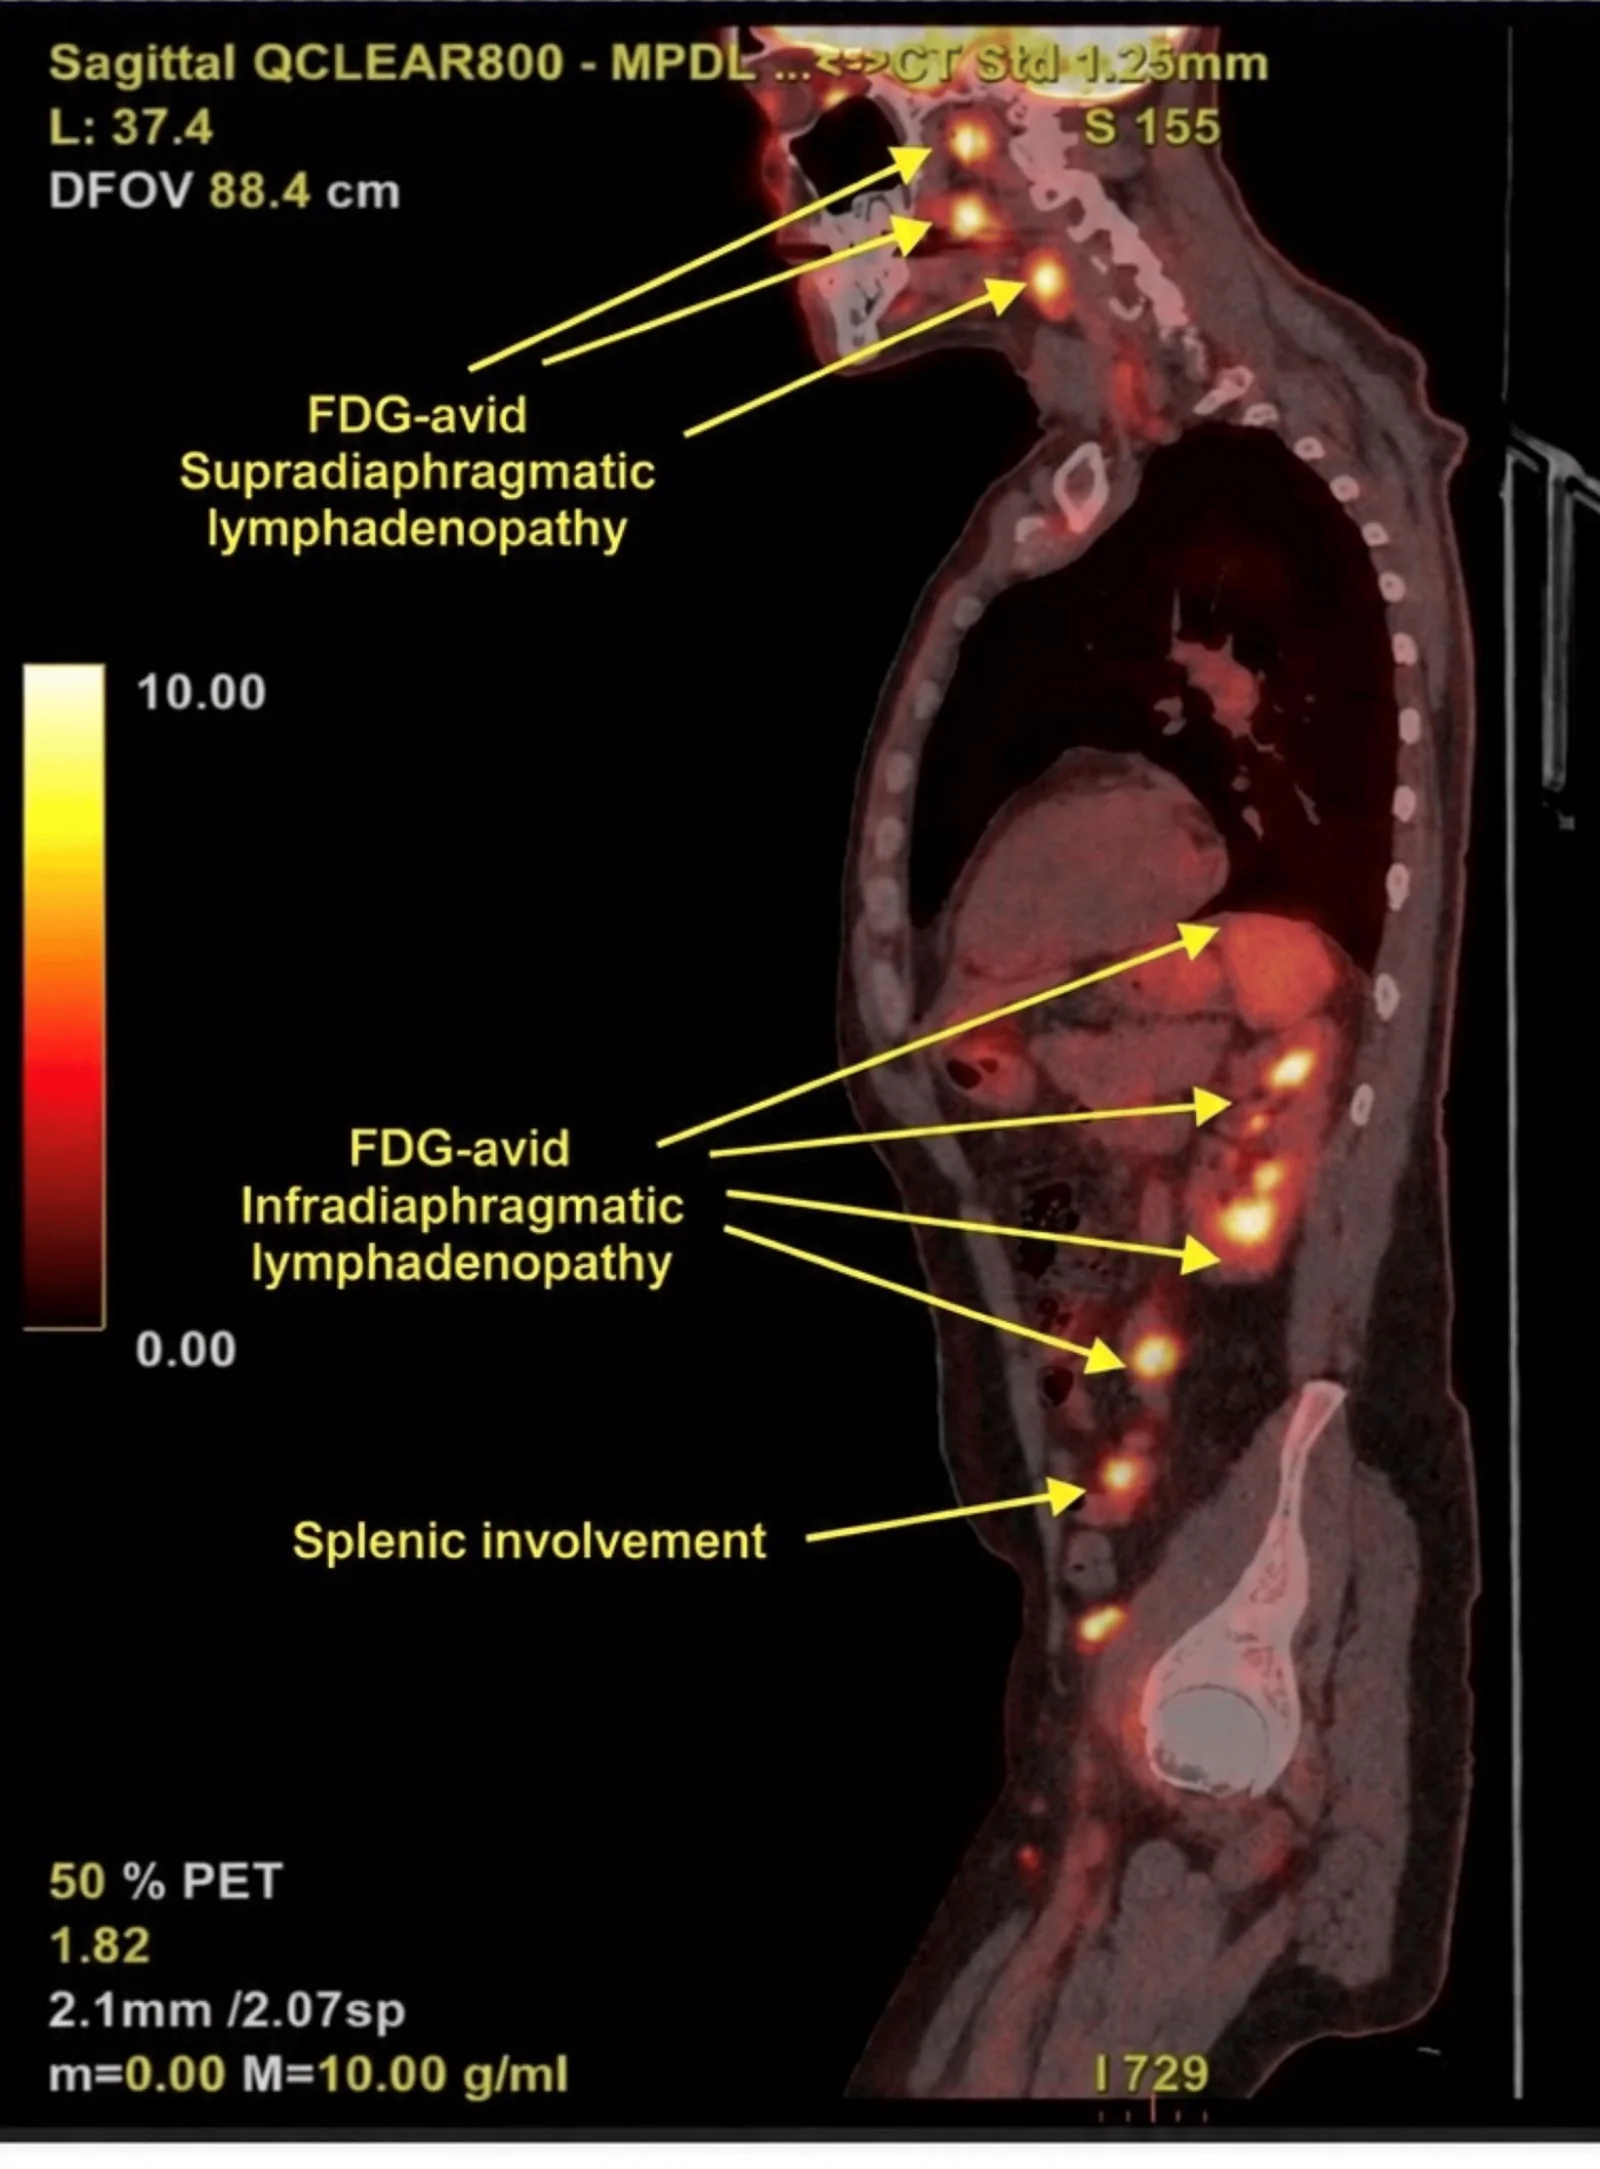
FDG PET-CT demonstrating metabolically active supra- and infradiaphragmatic lymphadenopathy with splenic uptake suggestive of lymphoproliferative disease PET: positron emission tomography, CT: computed tomography, FDG: fluorodeoxyglucose
